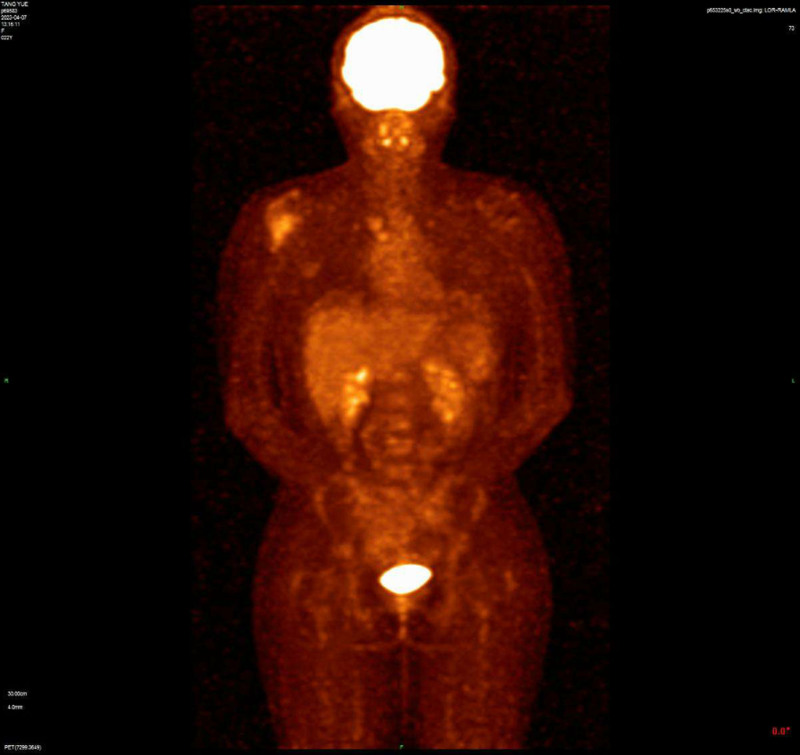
PET-CT revealed the presence of a tumor in the right proximal humerus and end of the clavicle. PET-CT = positron emission tomography–computed tomography.
